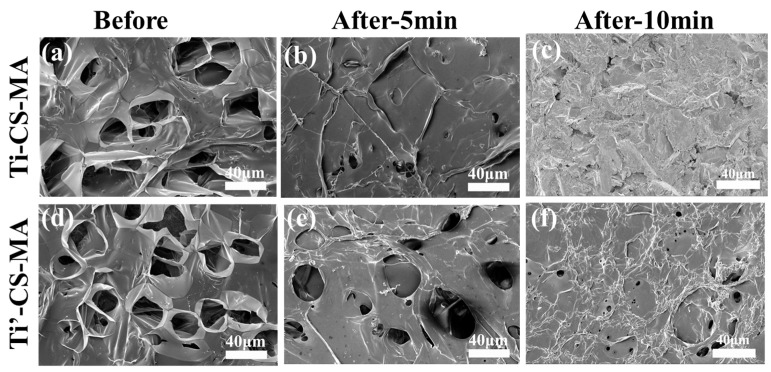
(a) SEM images of the cross-linked hydrogel layers that were covalent to the Ti surface (Ti-CS-MA,) before and after (b) exposure to ultrasonication (40 kHz, 80 W) for 5 min. After 10 min, the hydrogel layer was cut from the titanium surface, with only the pure titanium remaining (c). The hydrogel layers covalent to the Ti-TMSPMA substrates ((Ti’-CS-MA, (d)) maintained a good coating morphology after ultrasonication (40 kHz, 80 W) for 5 min (e), and no detectable damage was noted compared to non-TMSPMA-assembled titanium substrates. However, apparent damage was seen after exposure to ultrasonication (40 kHz, 80 W) for 10 min (f).
